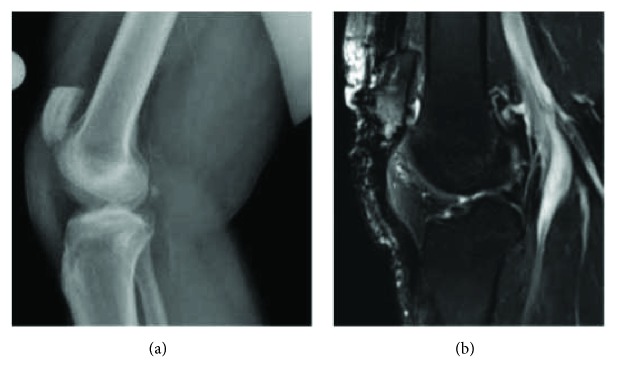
Lateral X-ray (a) and T2-weighted MRI (b) of the patient's right knee eleven months following the index procedure showing proximal migration of the patella and a redundant but intact patellar tendon. CDI = 1.51, ISI = 1.55.
